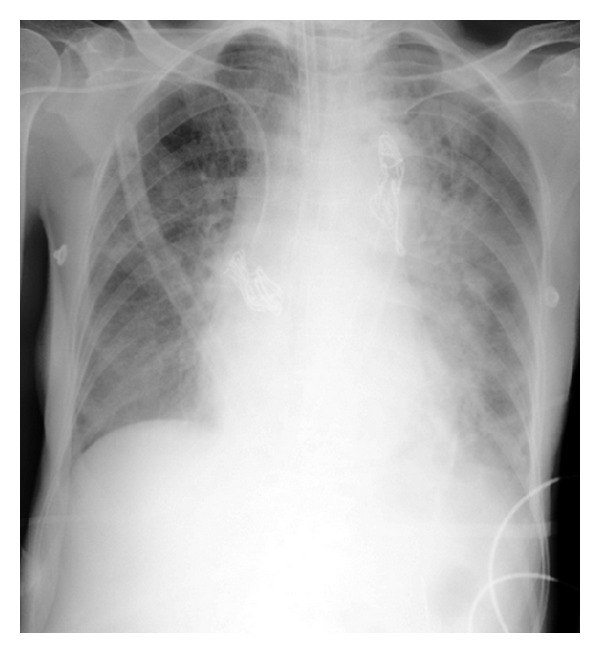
Chest X-ray showing a mass in the left upper lung field (Palla's sign).
